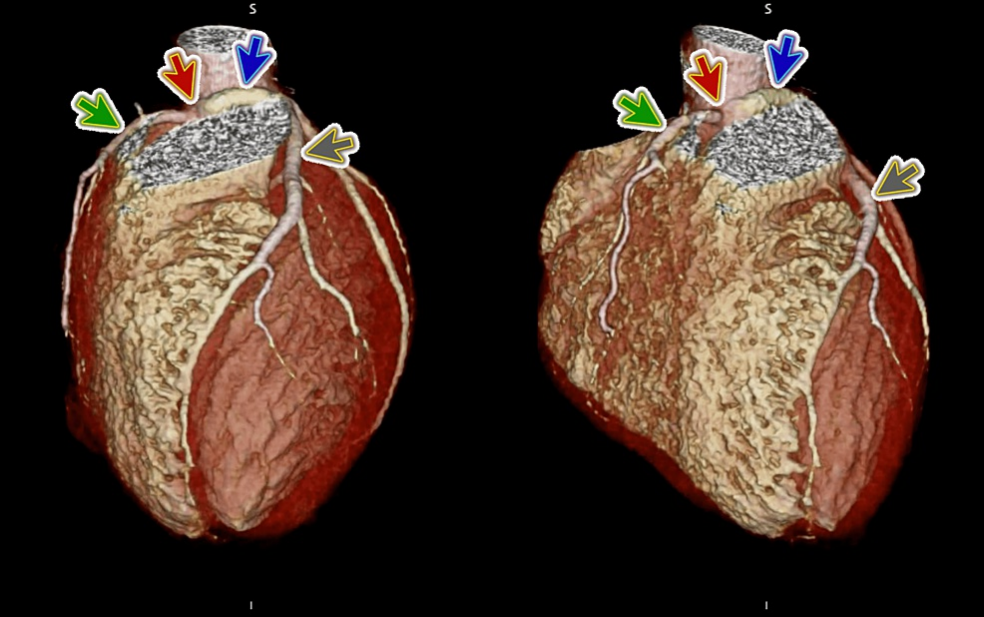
Computed tomography coronary angiography Computed tomography coronary angiography shows an anomalous origin of the left main coronary artery (blue arrow) arising from the right coronary sinus (red arrow); the grey arrow represents the left anterior descending coronary artery, and the green arrow represents the right coronary artery.
